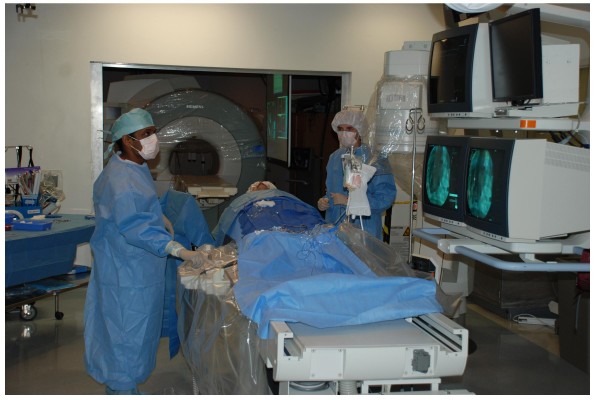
A combined X-ray and MRI interventional suite. The two systems can be used independently or together, when the radiofrequency-shielded and leaded doors are retracted. Patients can be transferred between labs on a cradle that slides along a motorized table. Each room can display real-time images, instantaneous hemodynamics, and imaging control to the operator. Both share a common control room for support staff [45].
